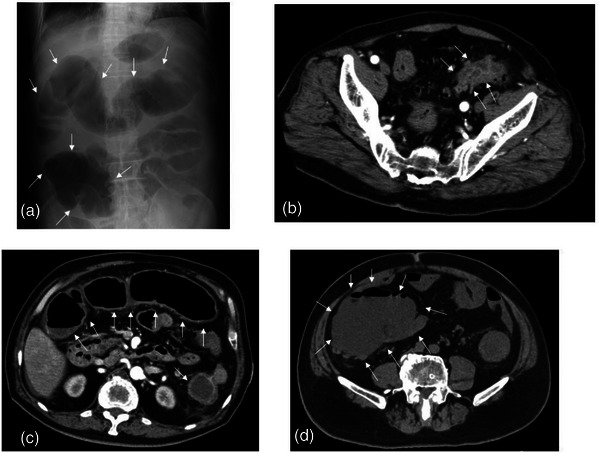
X‐ray and computed tomography images of obstructive colitis. (a) An X‐ray image showing severe colonic dilation from the cecum to the transverse colon. (b–d) Computed tomography images. (b) Obstruction owing to diverticulosis of the sigmoid colon. (c) Enhanced computed tomography image showing maintained blood flow to the colonic wall. (d) Severe dilation of the cecum (>10 cm).
